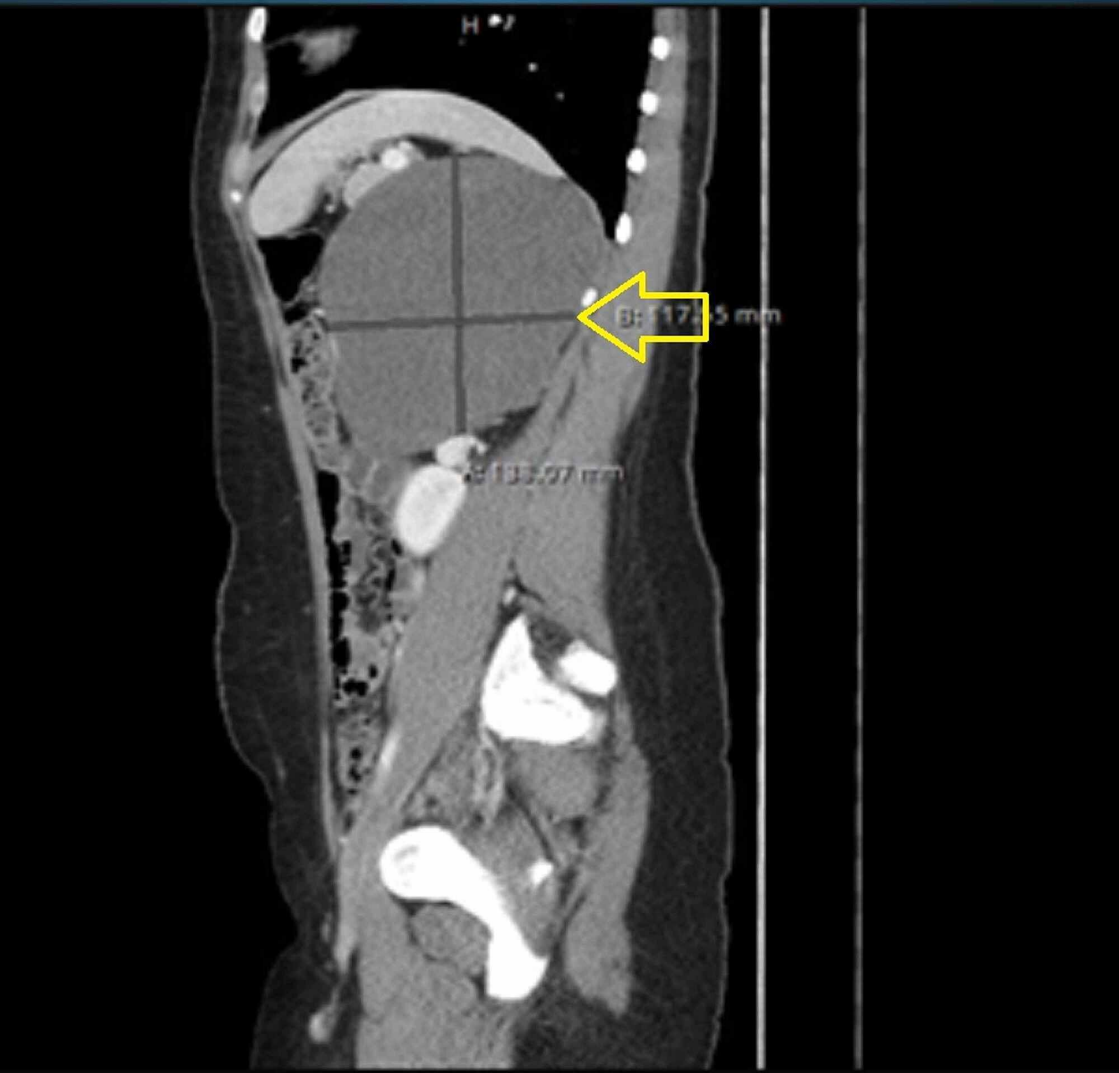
CT abdomen/pelvis sagittal view. The yellow arrow demonstrates a large suprarenal mass causing displacement of adjacent structures.
